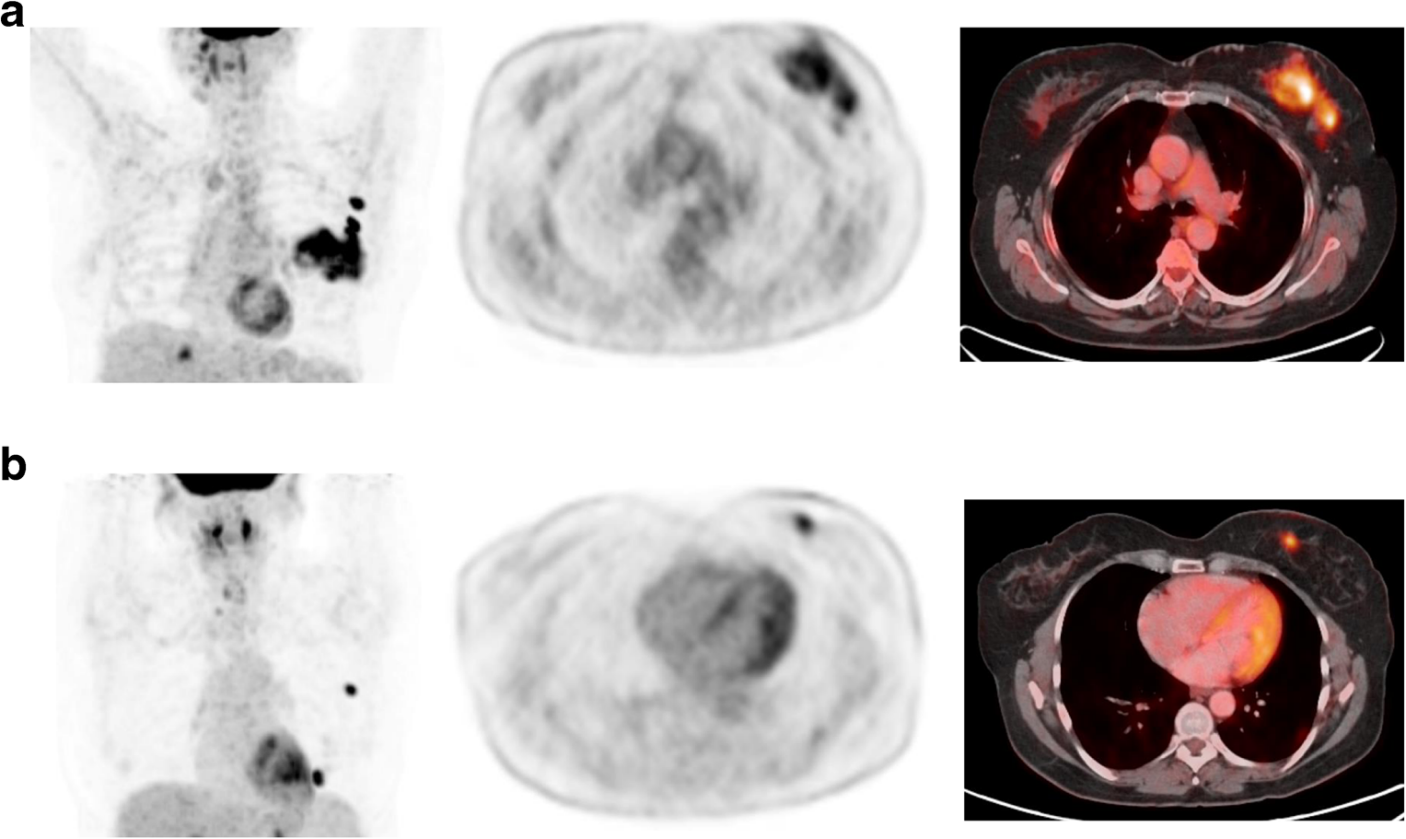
Two patients with breast cancer (a and b) with similar SUVmax but very different primary tumour MTV and TLG. a SUVmax 8.8, MTV 96.5, TLG 396.65; b SUVmax 8.5, MTV 2.7, TLG 10.8
